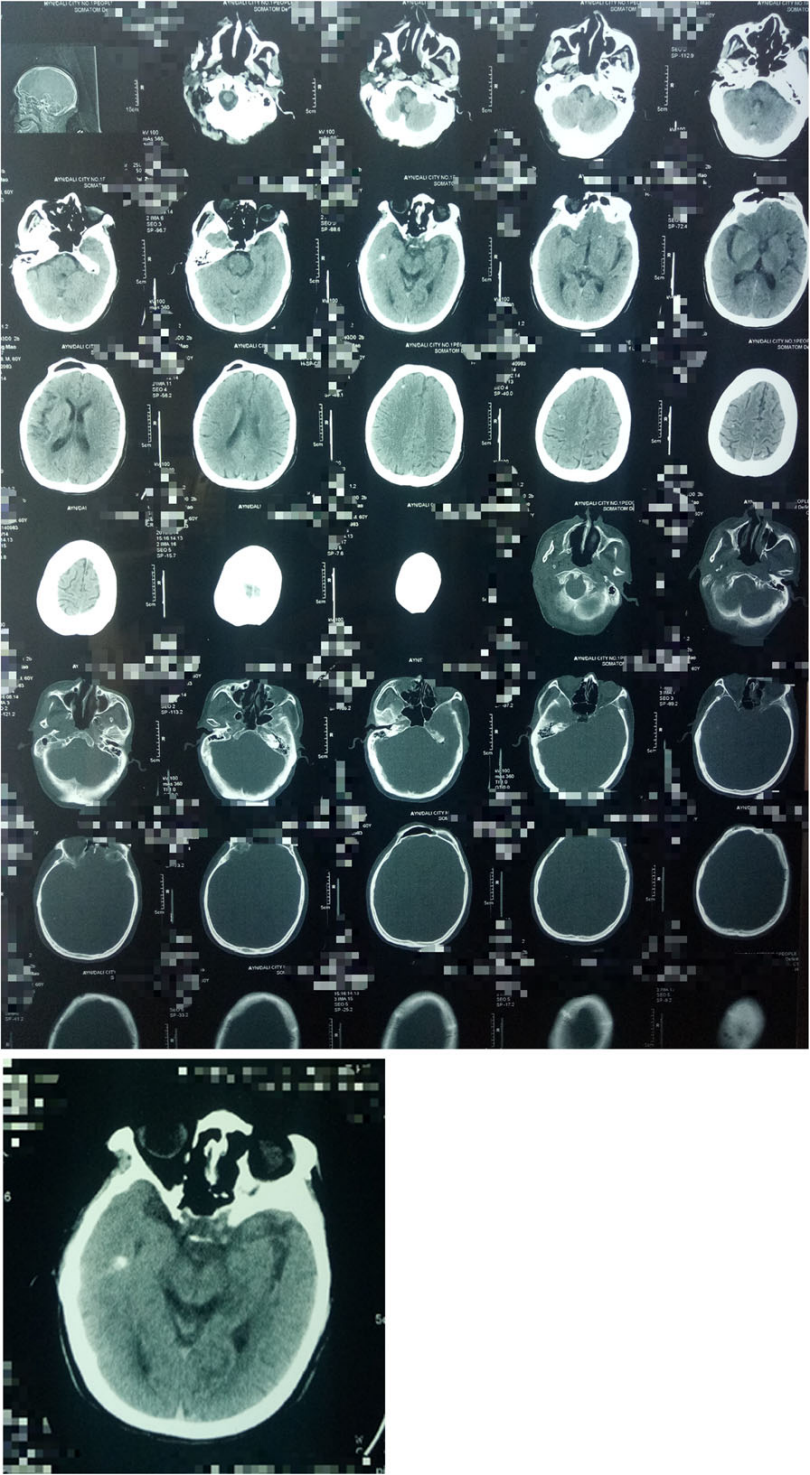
Brain CT scan (Bilateral nodules with multiple nodular calcifications were shown in bilateral cerebral hemispheres, with a diameter of approximately 1–2 mm)
